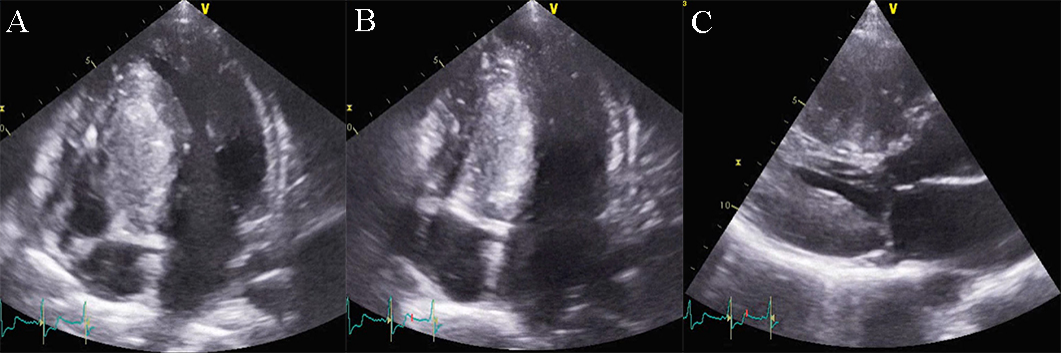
Echocardiographic aspect of HCM. (A) Apical 4 chamber view
